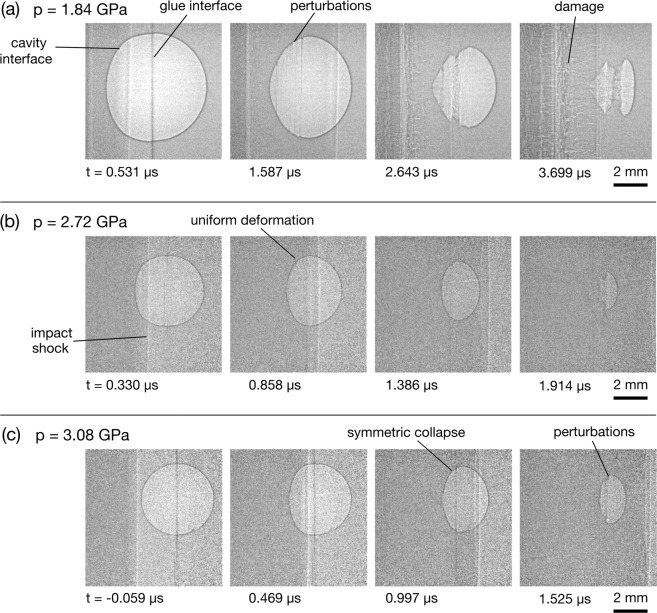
Radiograph sequences showing shock-cavity interactions in the transition regime. (a) A 6 mm cavity and 1.84 GPa shock. (b) A 4 mm cavity and 2.72 GPa shock. (c) A 4 mm cavity and 3.08 GPa shock.
